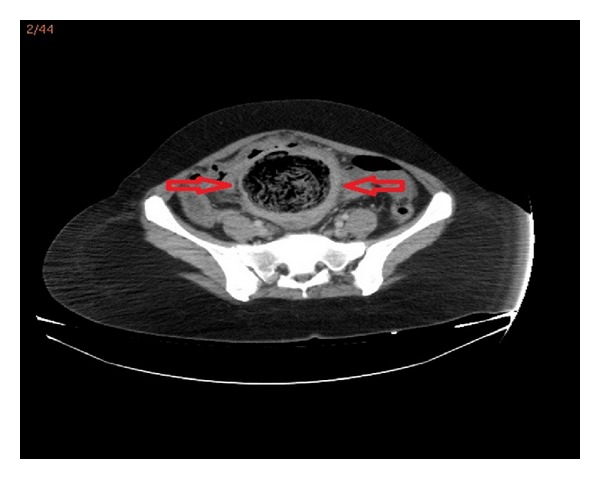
Abdominal CT scan revealed intra-abdominal mass—gossypiboma.
